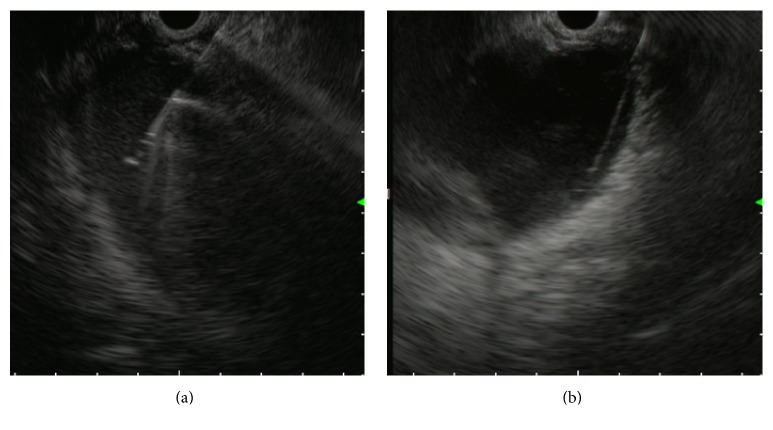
Endoscopic ultrasound (EUS) images. (a) EUS-guided puncture of a complicated cyst with a 19-gauge fine needle, and (b) placement of a 0.035-inch guidewire into the cavity.
